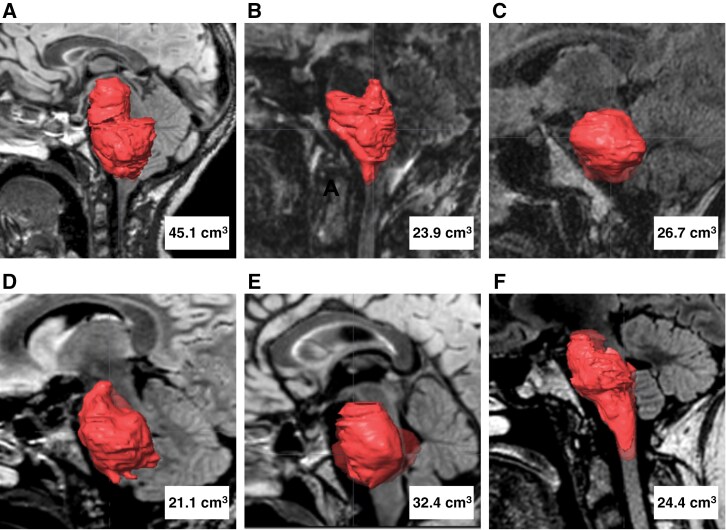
Tumor volumetric study. Tumor volume in cubic centimeters (cm^3^) based on FLAIR imaging prior to treatment for A) PNOC009-1, B) PNOC009-2, C) PNOC009-3, D) PNOC009-4, E) PNOC009-5, F) PNOC009-6.
